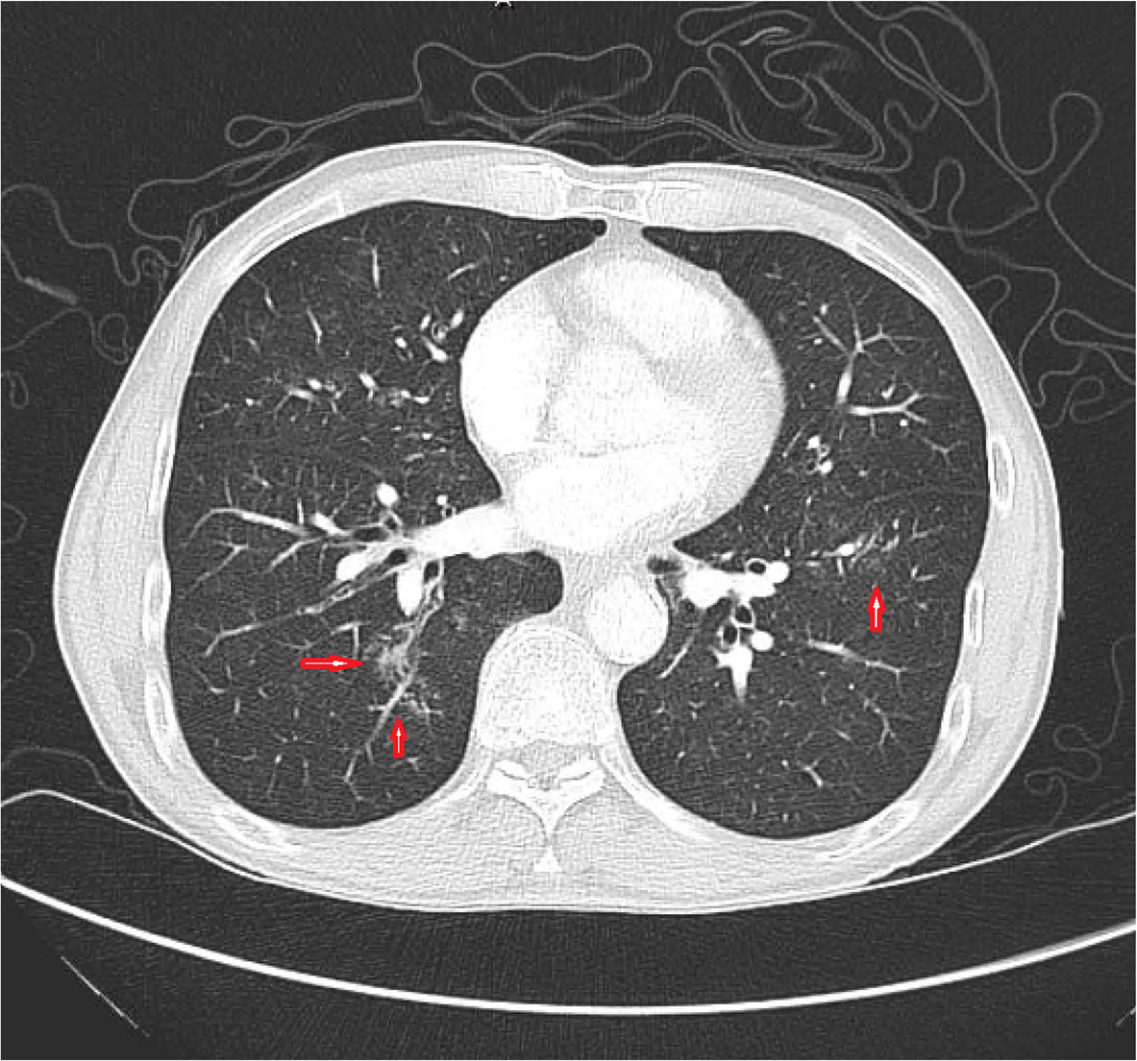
Computed tomography of chest revealing centrally located ill-defined ground-glass and mixed density nodular opacities greater on the right side
